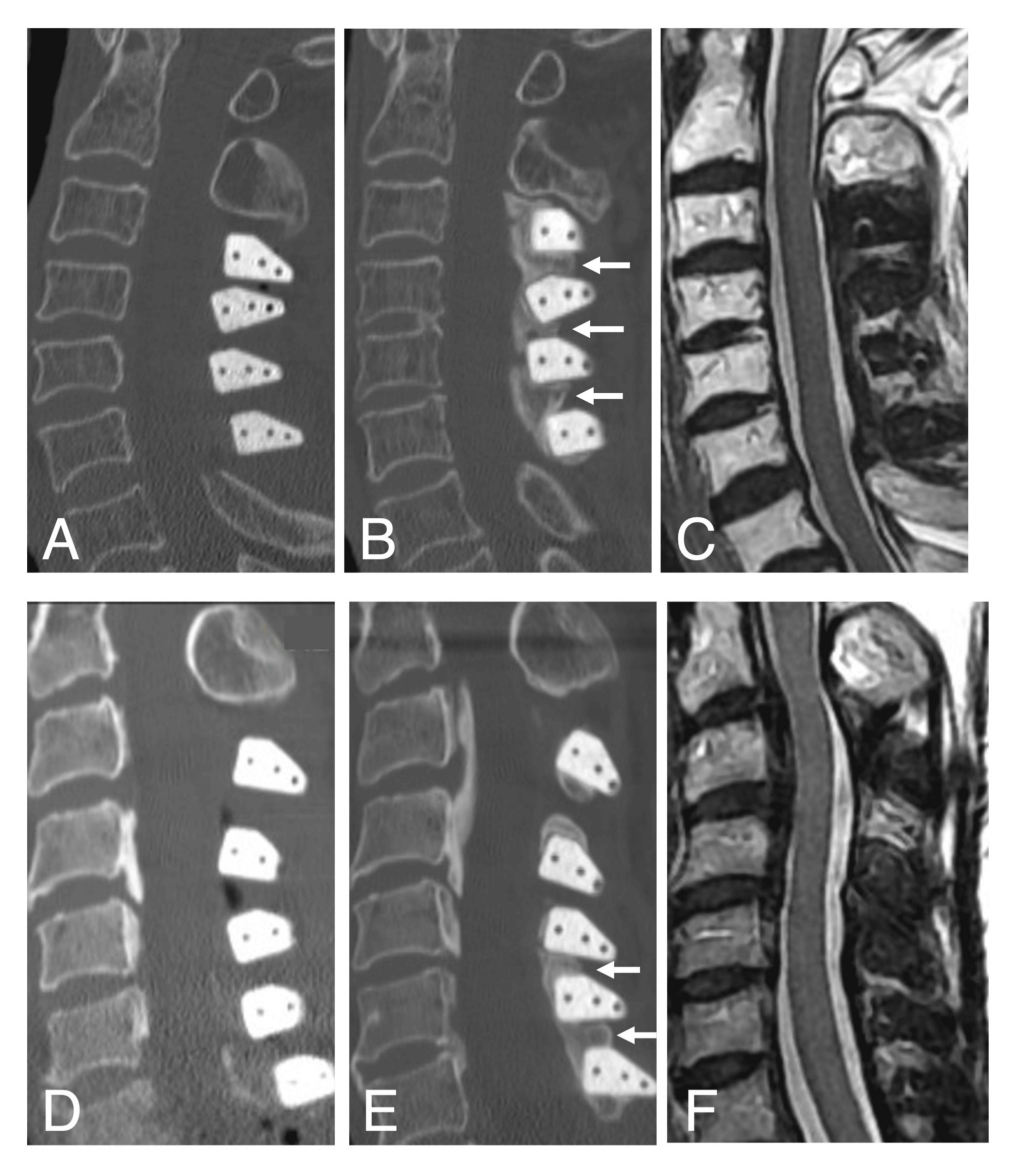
CT and MRI images of the cervical spine in cases of bone bridge formation Sagittal CT images of the cervical spine in patients with spondylotic myelopathy and OPLL immediately after surgery (A, D) and 52 and 93 months after surgery (B, E), respectively, demonstrating remarkable new bone bridge formation between adjacent hydroxyapatite spacers after the long-term postoperative period (white arrows). Sagittal MRI T2-weighted images of the same patients with spondylotic myelopathy and OPLL at 93 and 92 months after surgery, respectively (C, F), demonstrating that the subarachnoid space was well preserved. CT: computed tomography: MRI: magnetic resonance imaging, OPLL: ossification of the posterior longitudinal ligament
